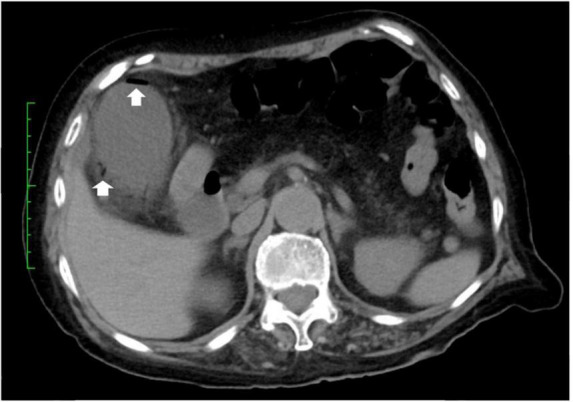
CT-scan of the abdomen. Abdominal CT showing air in the walls (arrows) of the gallbladder.
